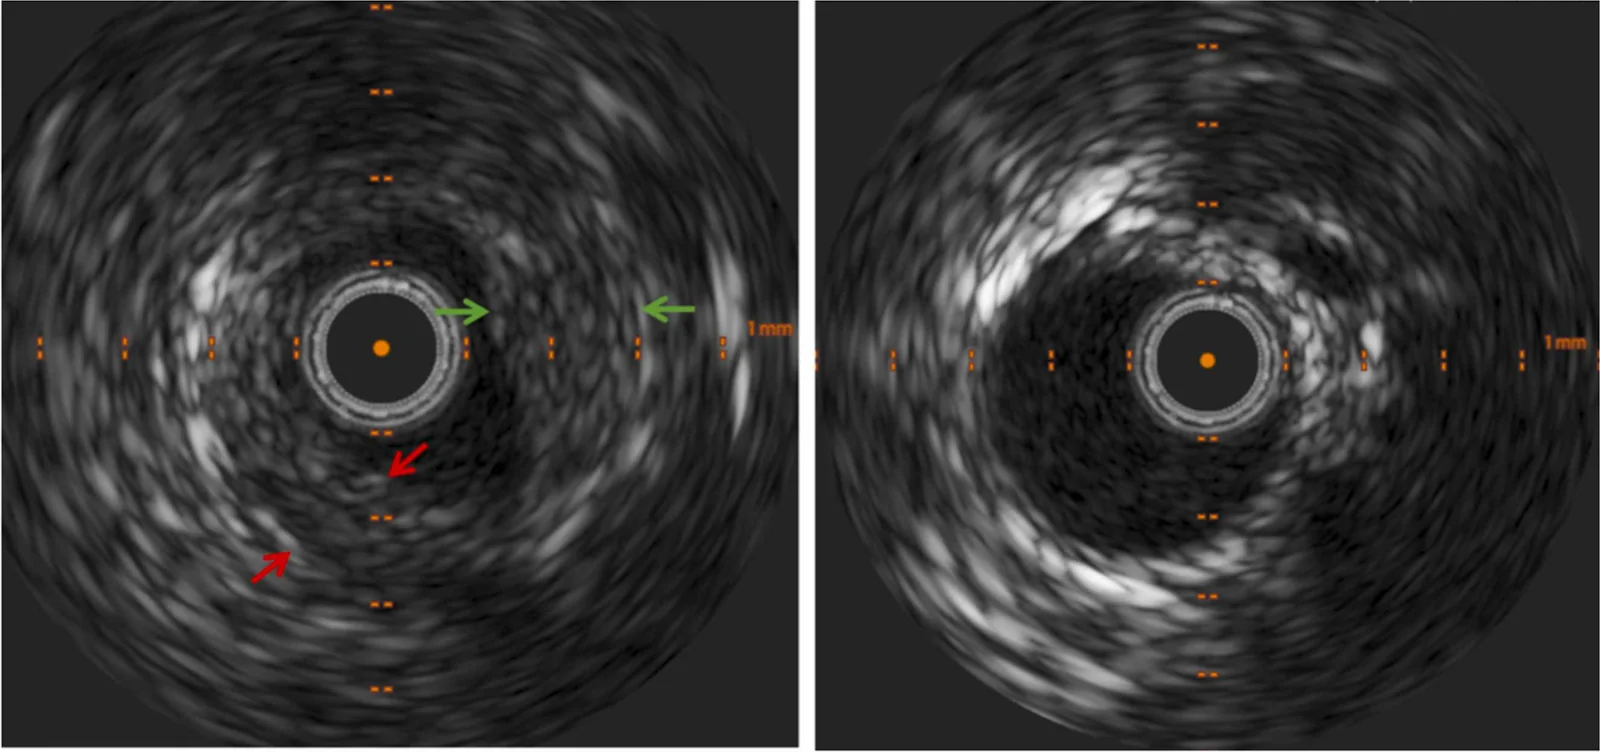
Intravascular ultrasound reveals intraluminal narrowing of the pulmonary vein. Intraluminal thickening (left) is highlighted with red and green arrows, obtained with IVUS 34 months post-transplant. A healthy pulmonary vein (right) is shown for comparison.
